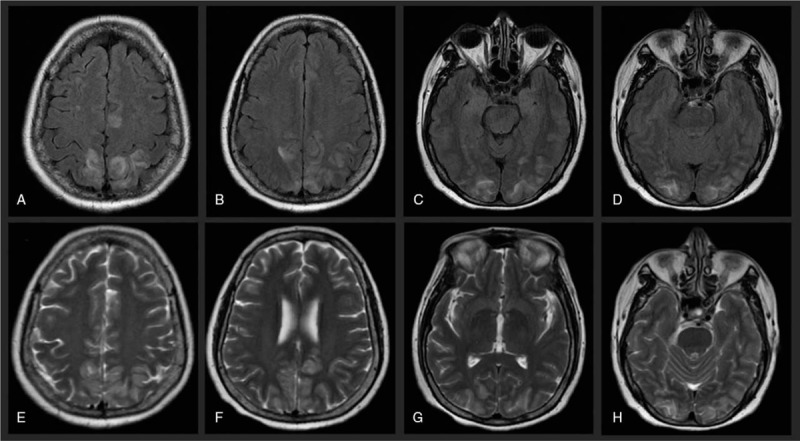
Case 1, MRI showed parieto-occipital hyperintensities on FLAIR (A–D) and T2-WI (E–H).
